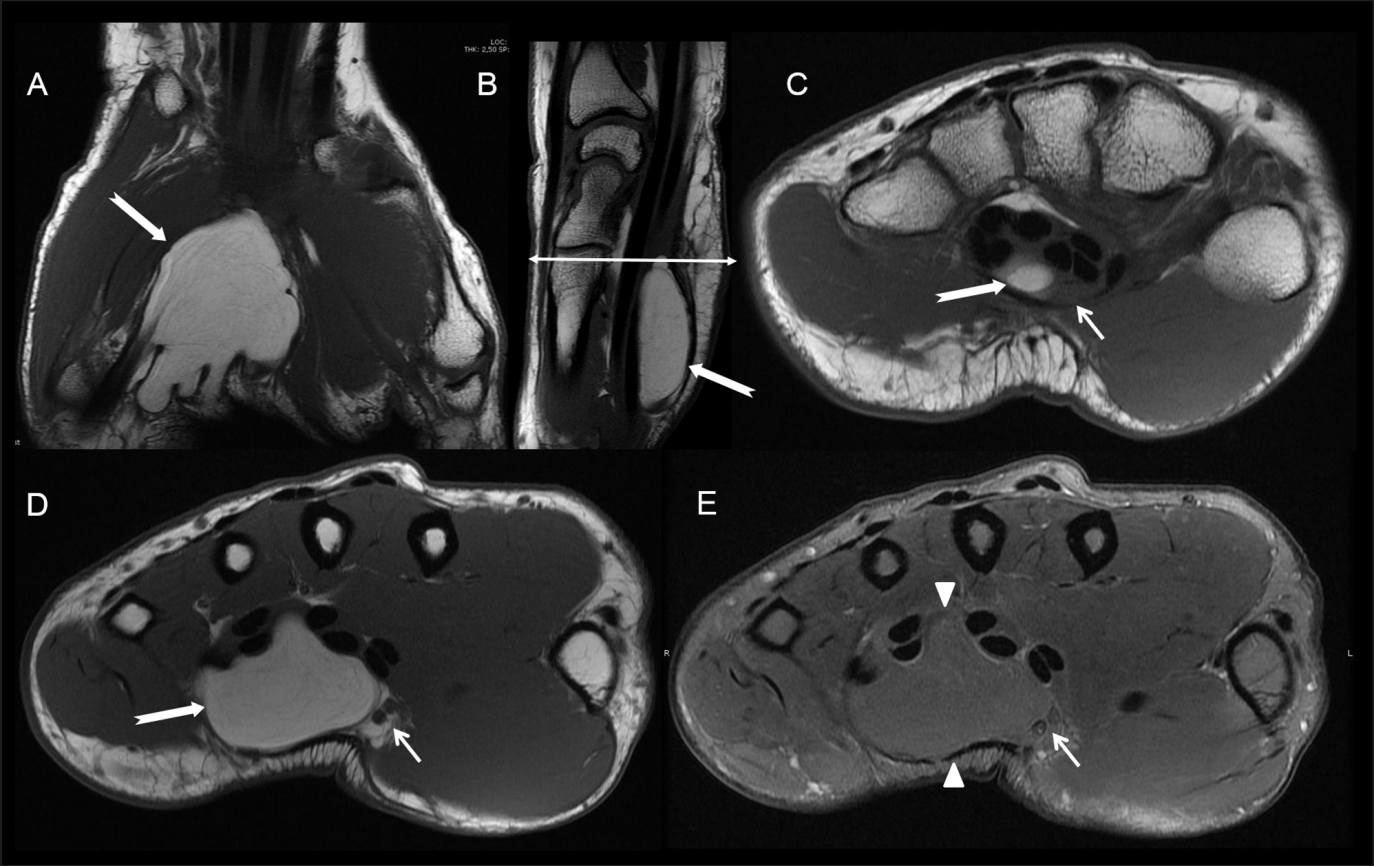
Carpal tunnel syndrome caused by a lipoma. MRI with T1-weighted coronal A), sagittal B) and axial (C, D) images and with T2-weighted FS axial E) image. MRI showed a space-occupying mass in the distal carpal tunnel with fat-like signal (increased signal in T1-weighted images (white arrows) with saturation on T2 FS image (arrowheads)) causing a compression of the median nerve and its branches (small white arrows).
